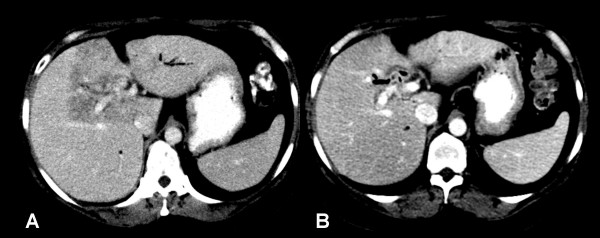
(A) Contrast-enhanced CT scan performed three months after completion of chemoradiotherapy. The arrows highlight the linear margins of the region of low attenuation. (B) CT obtained 9 months after radiation treatment. Oral and IV contrast were administered. The previously observed low attenuation region has resolved.
